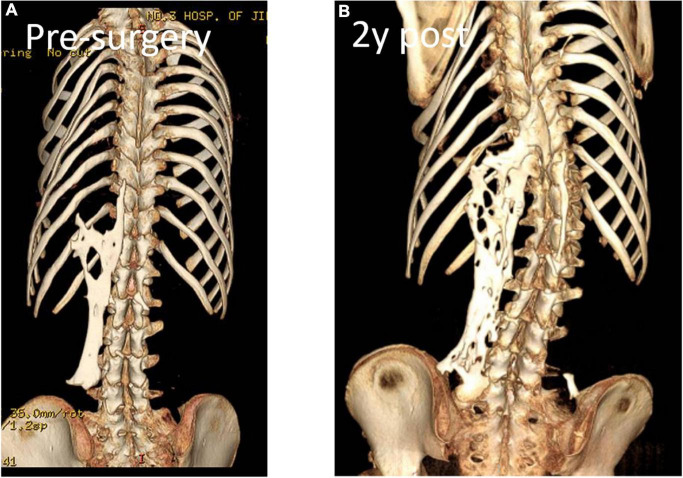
Computed tomography revealed spontaneous cervical fusion, heterotopic ossification of the left dorsal fascia causing scoliosis, and developmental deformity of the right chest wall.
